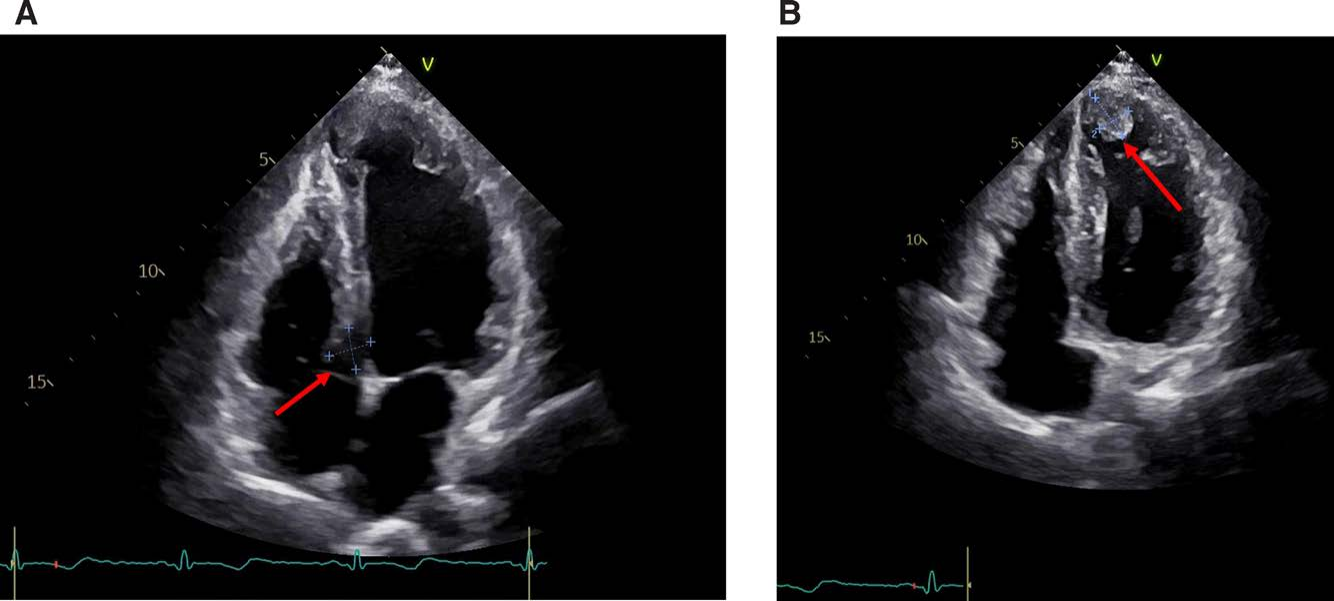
Echocardiography. (A) A membranous septal aneurysm (arrow) protruding into the right ventricular outflow tract with some hypoechoic tissue visible within it, which was considered to be a thrombus. (B) Left ventricular apical thrombus (arrow).
